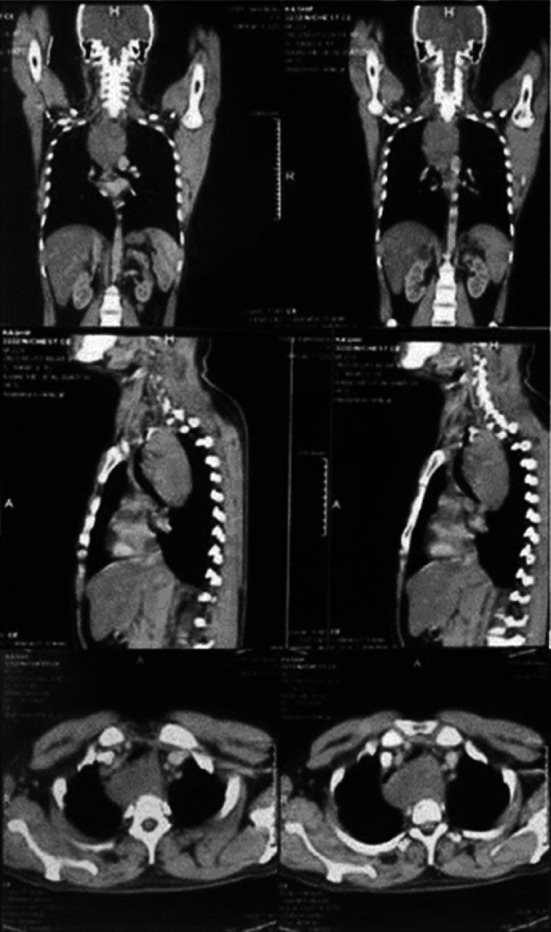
Computed Tomography Scan with contrast.
